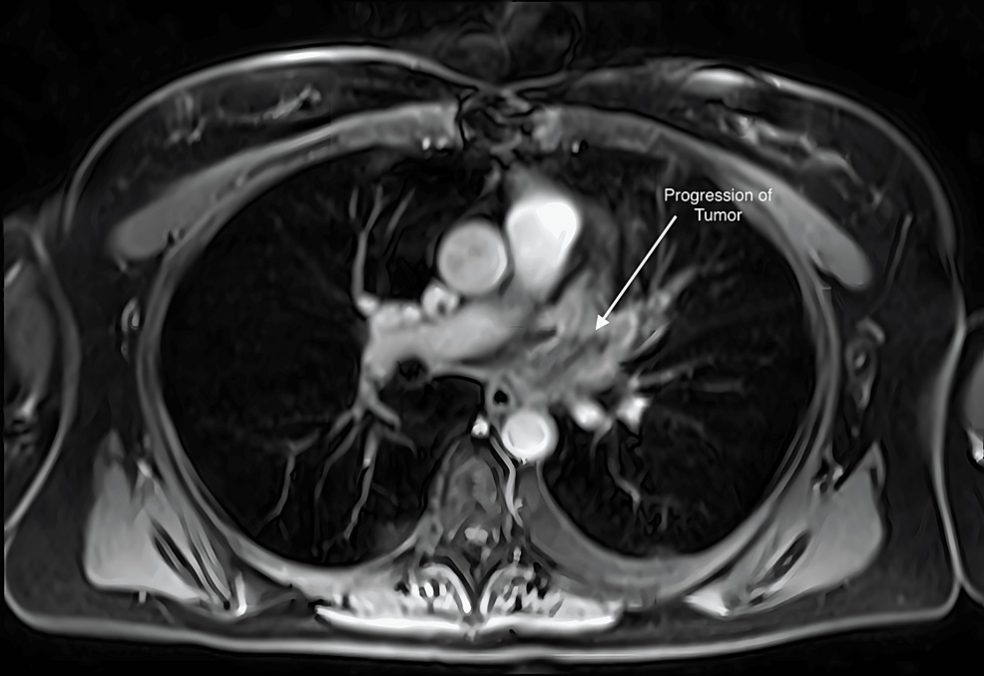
Cardiac MRI at four weeks demonstrating greater protrusion into the left atrium and new partial impediment of the inflow of the left lower pulmonary vein. The component of the mass at the level of the left atrium measured 4.0 cm × 2.7 cm.
